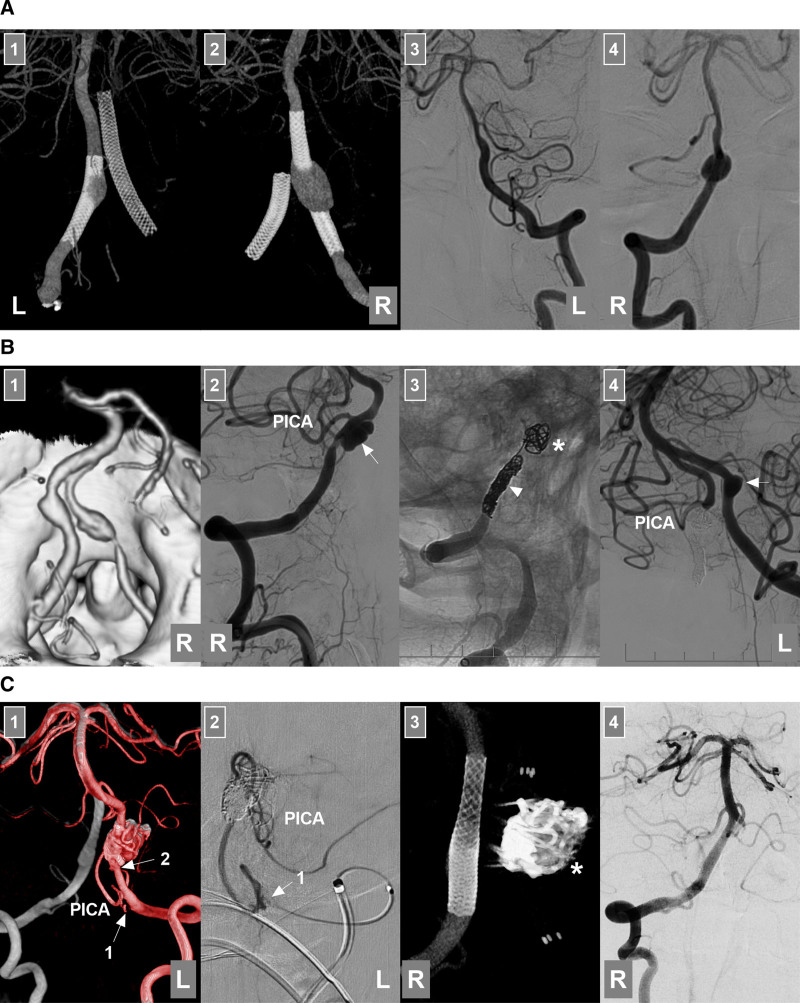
EVT of bilateral intracranial VA dissecting aneurysms. (A) FD deployment for bilateral VA dissecting aneurysms: panels 1 and 2: vaso-reconstructive DSA images showing 2 VA aneurysms treated with FD deployment; panel 3 and 4: 1-year follow-up DSA image showing cure of the left but not the right aneurysm. (B) PAO for ipsilateral ruptured VA dissecting aneurysms: panel 1: computed tomography angiography image showing bilateral VA dissecting aneurysms; panel 2: DSA image showing a right VA dissecting aneurysm (arrow) at the PICA origin; panel 3: Unsubtracted DSA image showing loose coiling of the right aneurysm (asterisk) and complete occlusion of the proximal VA (arrowhead); panel 4: DSA image showing a right PICA supplied retrogradely by the left VA; plans were made to treat the left aneurysm (arrow) later. (C) Simultaneous deconstructive and reconstructive EVT: panel 1: reconstructive 3-dimensional DSA image showing bilateral VA dissecting aneurysms, the left of which recurred after coiling assisted by traditional stenting, while the left PICA has a double origin (Arrows with numbers 1 and 2); panel 2: microcatheter angiography in the lower origin of the PICA (arrow with number 1) showing the course of the vessel; panel 3: vaso-reconstructive DSA image showing coil occlusion of the left aneurysm (asterisk) and FD deployment to cover the right aneurysm; panel 4: 6-month follow-up DSA image of the right VA showing cure of the right aneurysm and sufficient blood flow to the vertebrobasilar arteries from the right VA. DSA = digital subtraction angiography, EVT = endovascular treatment, FD = flow diverter, L = left, PAO = parent artery occlusion, PICA = posterior inferior cerebellar artery, R = right, VA = vertebral artery.
